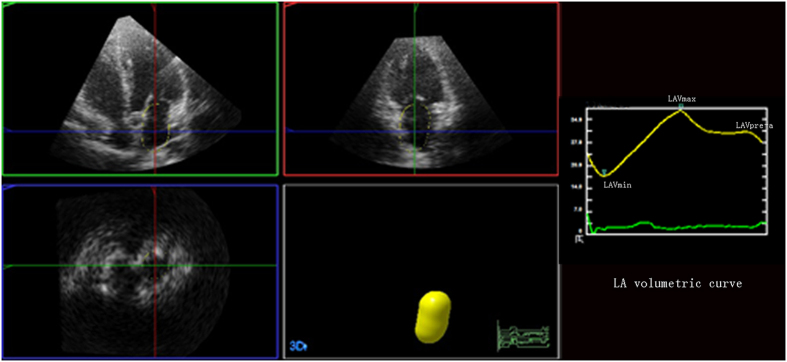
Example of one three-dimensional echocardiography study. LAVmax (maximum left atrial volume), LAVmin (minimal left atrial volume), LAVpre-a(preatrial left atrial volume).
